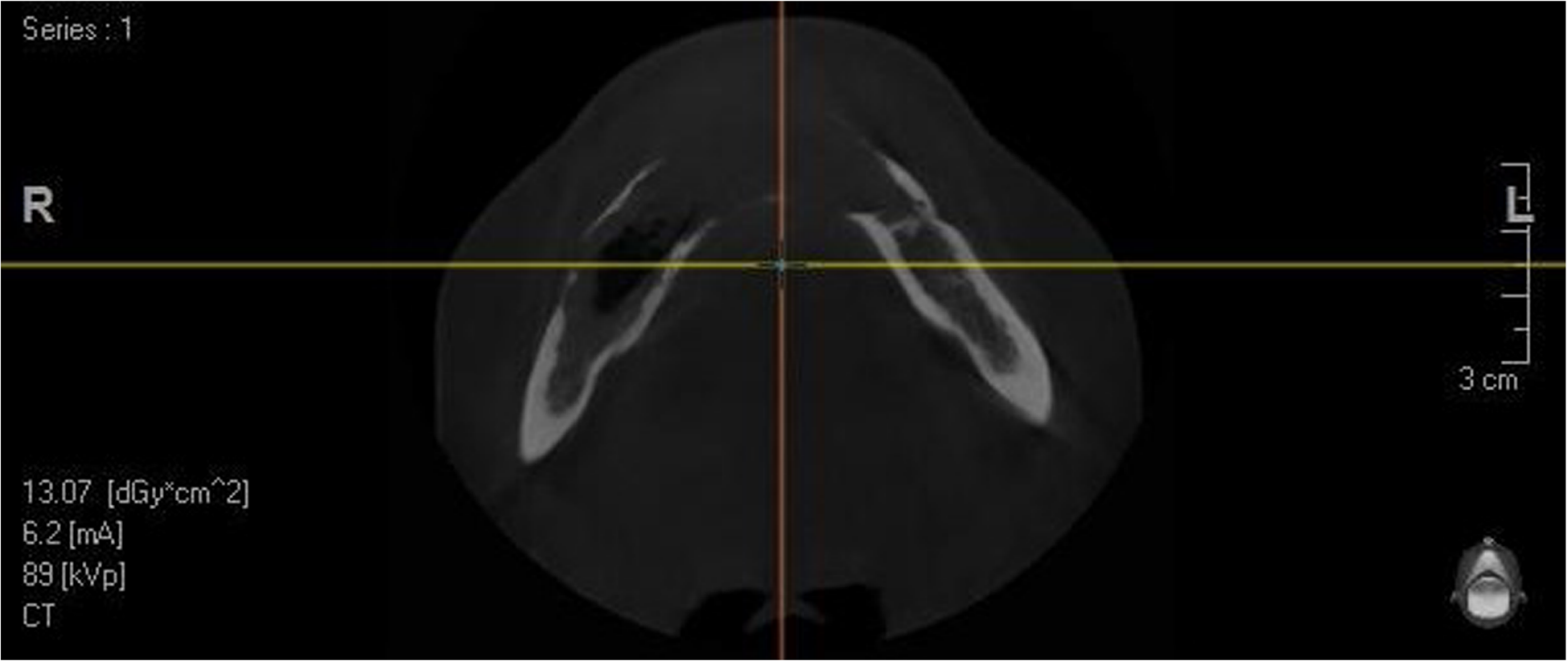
Cone beam computed tomography of the mandible. Axial view of lesion
